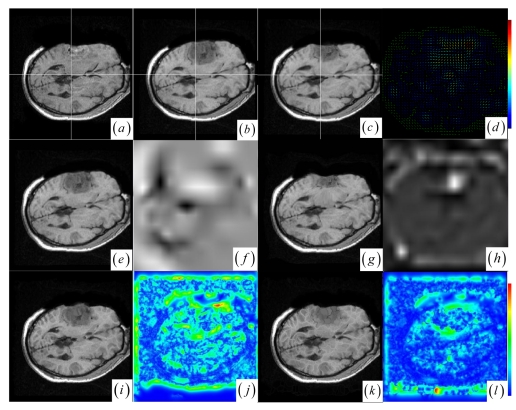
(a)–(b) Intra- and pre-operative MR images. (c) JKC. (d) Displacement vector field with the vector orientation and the variations of the vector color (the color scale encodes the norm of the displacement vector, in pixels). (e) BMI. (f) BMI deformation image. (g) BCR. (h) BCR deformation image. (i) DEM. (j) DEM deformation image (the color scale encodes the norm of the displacement vector, in pixels). (k) DIF. (l) DIF deformation image.
